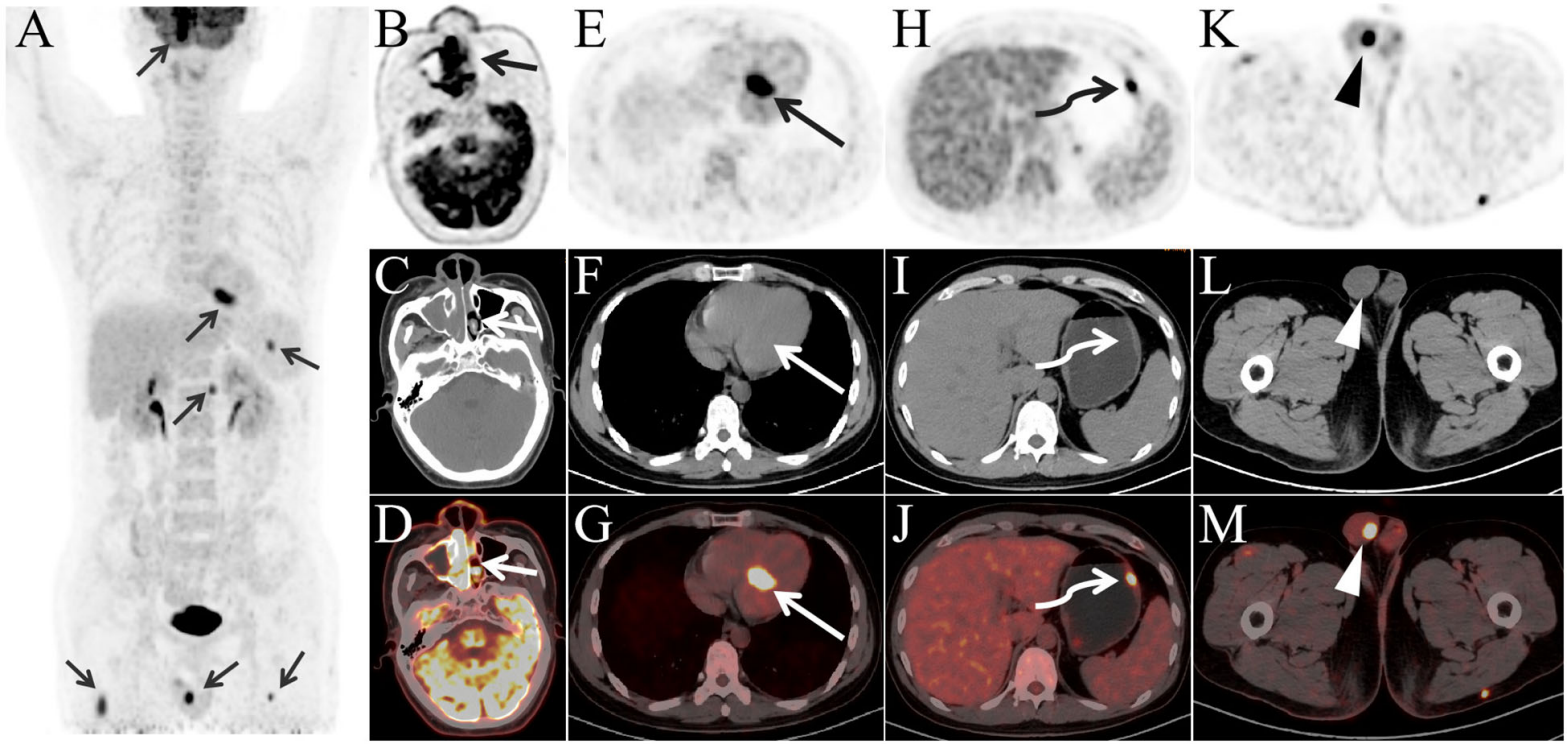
The maximum intensity projection image (A) reveals multiple FDG-avid lesions (arrow) throughout the body. Panels (B–D) depict soft tissue in the bilateral nasal cavity and right maxillary sinus (short arrow) with significantly increased FDG uptake, SUVmax 16.9. Panels (E–G) show an FDG-avid lesion in the left atrium (long arrow), SUVmax 14.6. Panels (H–J) demonstrate a mildly thickened gastric wall (curved arrow) with elevated FDG uptake, SUVmax 9.3, while panels (K–M) reveal a nodular FDG-avid lesion in the right testis (arrowhead), SUVmax 9.3.
